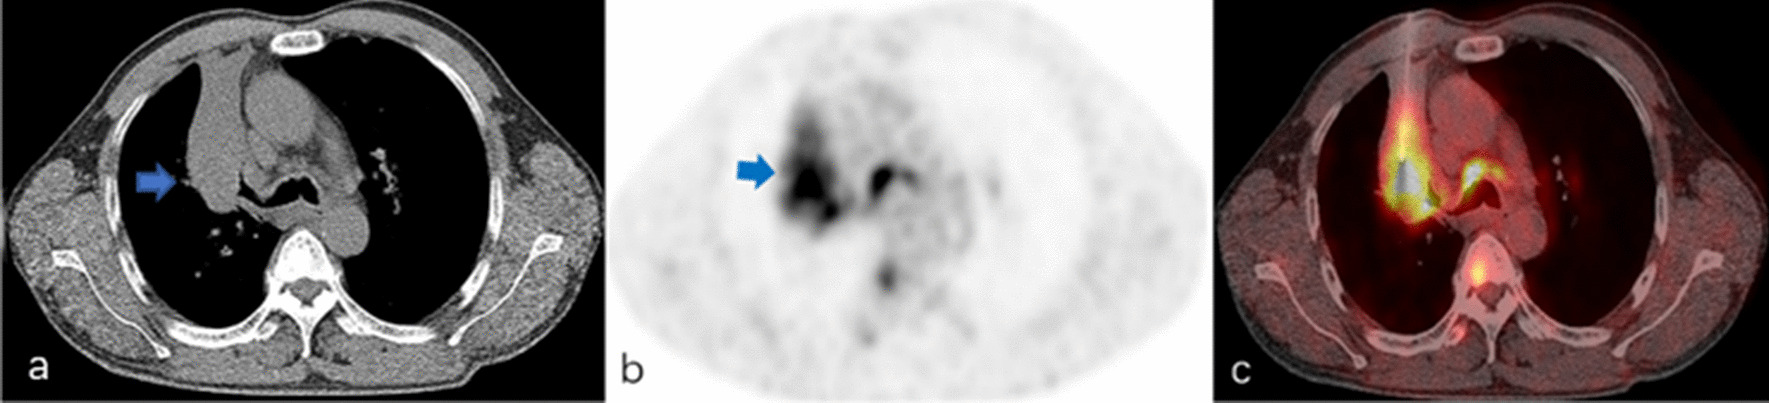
A 52-year-old man with suspected primary lung malignancy. a The intraprocedural non-contrast CT image (using mediastinal soft tissue window) showed a heterogenous mass in right upper lobe. b
